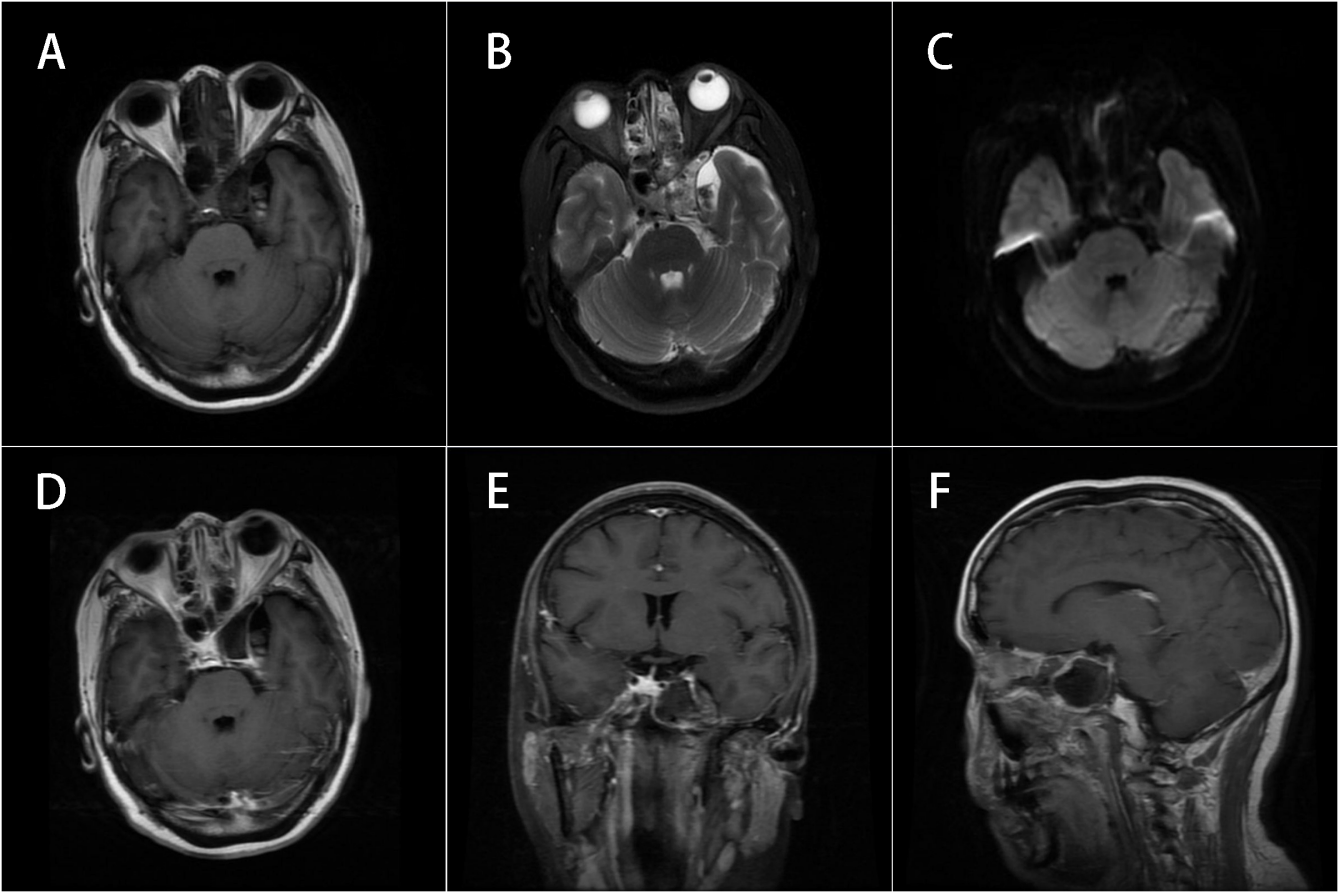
MRI of the brain with and without contrast. (A–C) Corresponded to Figure 1A–C and (D–F) corresponded to Figure 1E–G respectively. The lesion had been completely resected and the abnormal signals observed on the preoperative imaging had disappeared.
